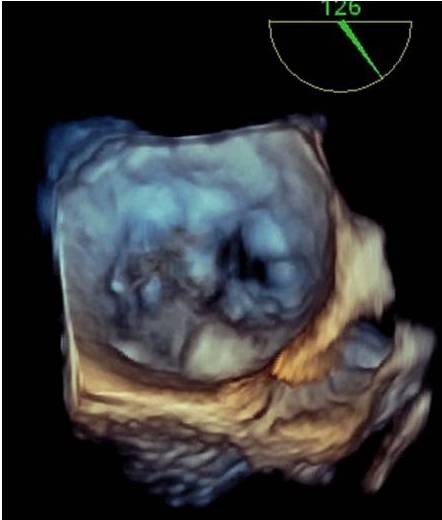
Echocardiographie tridimensionnelle en échographie transoesophagienne (ETO) misant en évidence l’anévrysme mycotique de la valve mitrale postérieure
